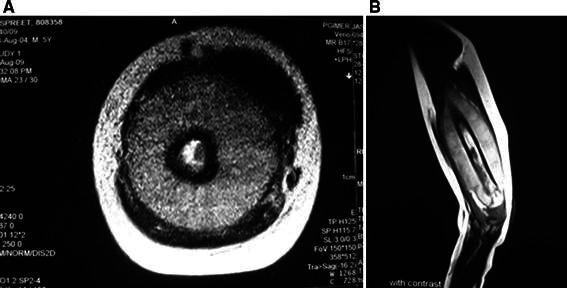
a Axial section at the mid-shaft humerus after gadolinium-enhanced MRI, showing circumferential, moderately enhancing, well-marginated peridiaphyseal soft-tissue mass. b Coronal section of the humerus after gadolinium-enhanced MRI showing an intramedullary altered signal with moderate enhancement involving almost the whole of the diaphysis
